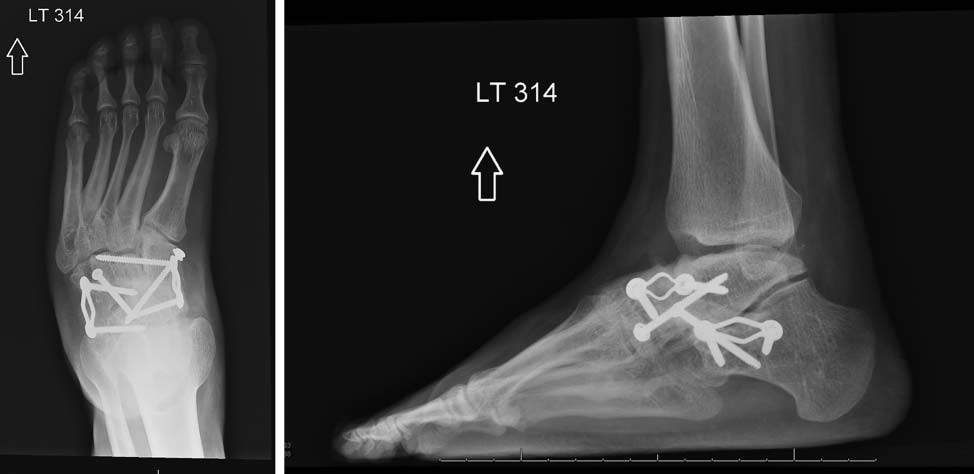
Anteroposterior and lateral radiographs 1 year after navicular excision, talocuneiform arthrodesis, and calcaneocuboid arthrodesis.
